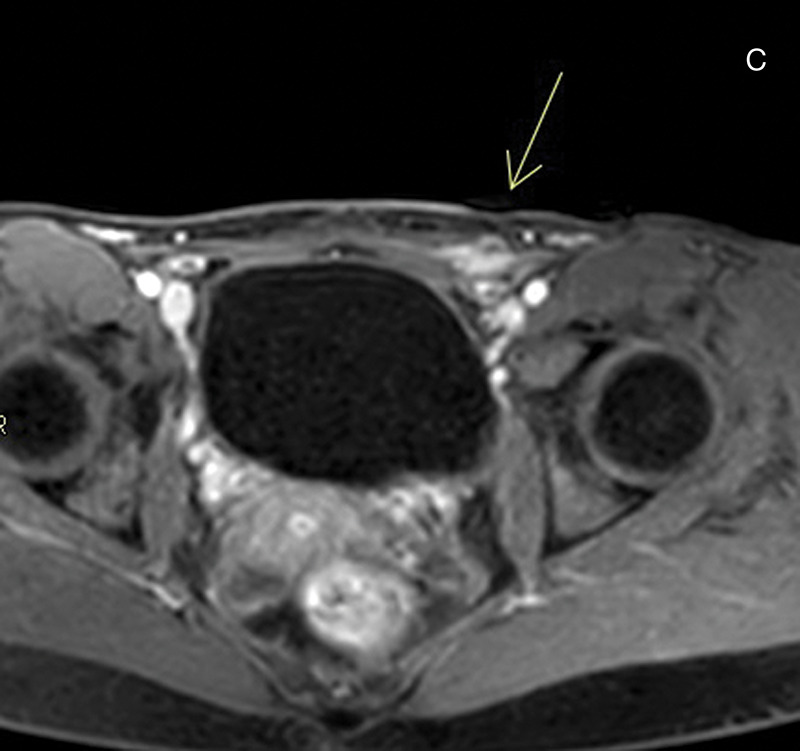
A. Ultrasound shows an mildly heterogeneous, hypoechoic mass with vascularity. B. Axial T1‑weighted fat suppressed image shows an isointense area in the left inguinal region. C. Same region as in Figure 1b, after intravenous administration of gadolinium, showing vivid lesional enhancement.
